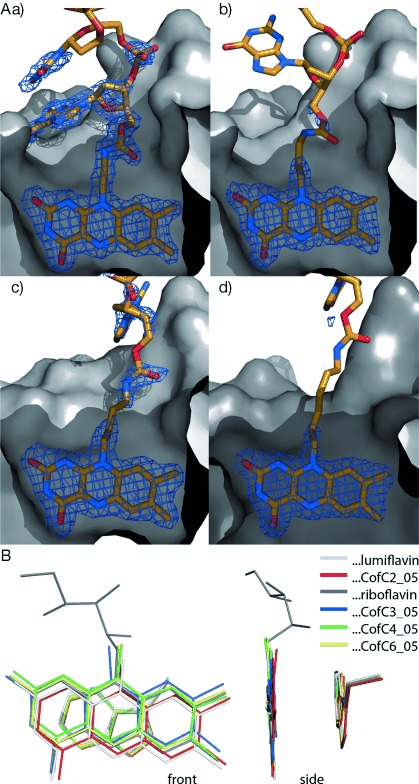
X-ray structures of CofC*n*_O5 flavin–DNA ligands in the tE dodecin binding pocket. Dodecin is depicted as apoprotein in a surface representation (gray) with the incorporated ligands shown in stick representation (C orange, N blue, O red). Dodecin has six binding pockets for the incorporation of dimers of flavins in a *C*_2_-symmetric manner. For clarity the *C*_2_-symmetric part of one binding pocket is shown. Structural data used for figures are deposited with the protein data bank (pdb) codes 2vkg, 2vkf, and 4b2h (http://www.rcsb.org). A) tE dodecin with bound CofC*n*_O5 ligands with varying lengths of alkyl chain connecting isoalloxazine to DNA. CofC2_O5 (a) has an ethyl chain, CofC3_O5 (b) propyl, CofC4_O5 (c) butyl, and CofC6_O5 (d) hexyl. Electron omit density is shown at *σ*=1.5 as a blue mesh, highlighting protein-bound substructures of ligands. The unbound parts of the ligands, which could not be identified by electron density, are shown for clarity in one possible conformation. (B) Superposition of Cα-backbone-aligned tE dodecins with CofC*n*_O5 ligands (and tryptophan, which is part of the binding site) in front and side view. Ligands are depicted by their isoalloxazine anchor. Wildtype dodecin with riboflavin and lumiflavin ligands are shown for comparison.
